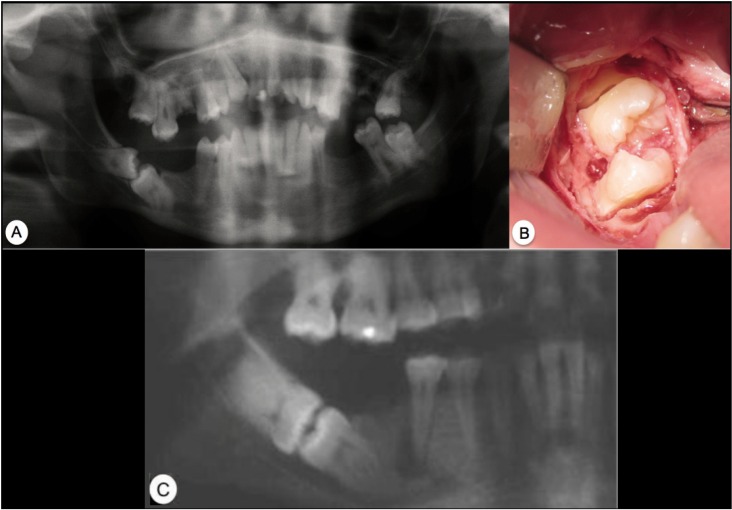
Case 3. A) Preoperative orthopantomogram. B) Observe the tissue flap, the ostectomy and tooth sections, separating the crown from the roots, just before being removed using forceps. C) Case 4. Preoperative orthopantomogram.
